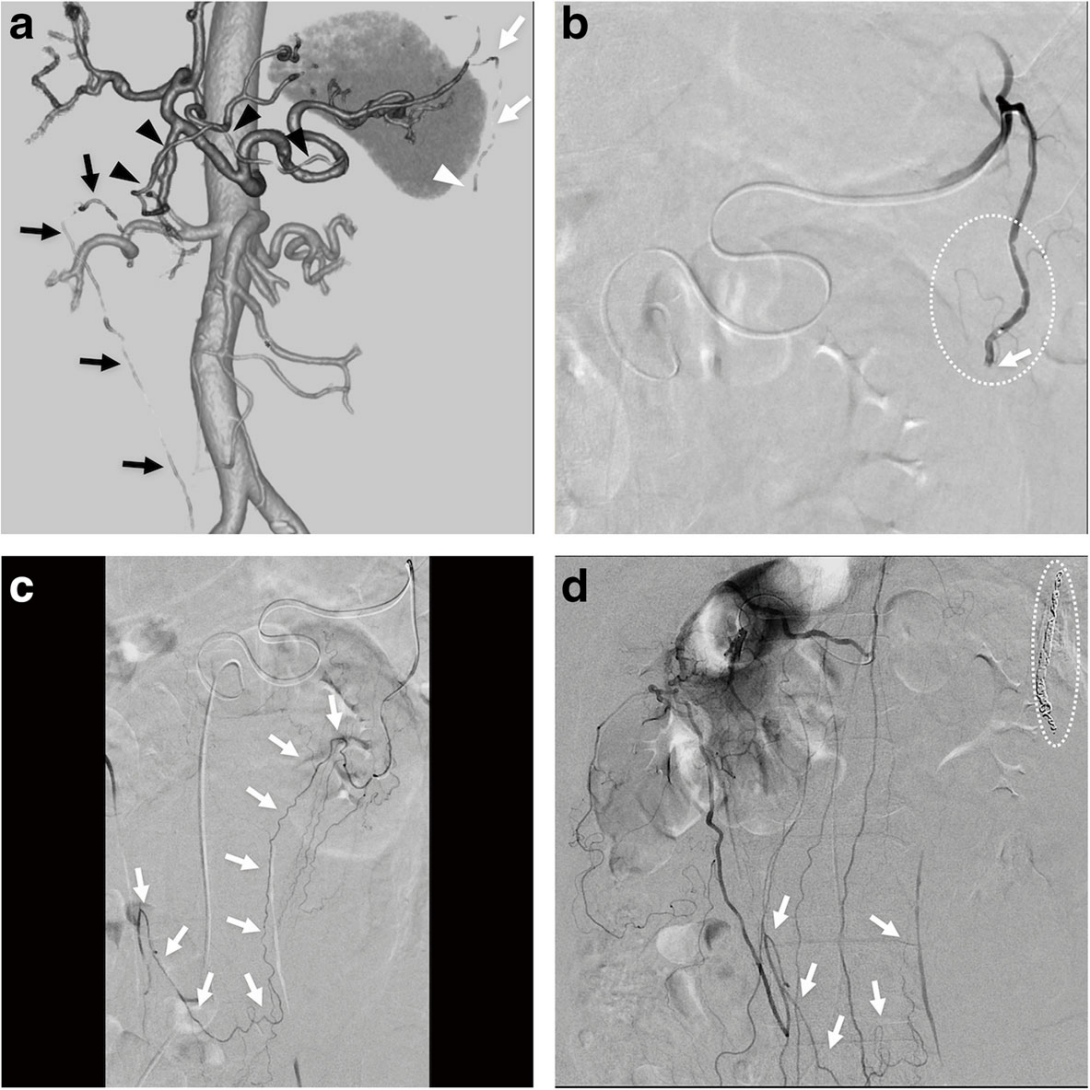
Images from a 60-year-old man with left OA bleeding associated with SAM. a Coronal volume-rendered computed tomography image in cranial and right-anterior projection reveals that a left OA (white arrows) arises acutely from a splenic artery branch and develops subtle dilation and stenotic change. The distal side of left OA is completely occluded (white arrowhead), presumably associated with SAM. Note that right gastroepiploic artery (black arrowheads) courses alongside the greater curvature of and one of the right omental branches off vertically (black arrows), which is found to be directly communicated with the to-be embolized left OA (white arrows). SAM is the most likely diagnosis, and transarterial embolization is planned. b DSA of left OA in right anterior oblique projection reveals that it acutely arises from a splenic artery and that it develops the dilation and stenotic change (dotted circle) and complete occlusion (arrow). Given that embolization of the proximal left OA at the arrow-indicating point could lead to incomplete hemostasis or rebleeding via arterial collateral network through another OA arising from right gastroepiploic artery, attempt was made to navigate the microcatheter into the distal side beyond the occluded point without complications. c DSA of the distal side of left OA in posteroanterior projection reveals that distal left OA does not develop findings suggestive of SAM. Note that the to-be-embolized left OA directly communicates with a right OA arising from right gastroepiploic artery (arrows). Embolization was successfully performed using isolation technique with coils. d DSA of gastroduodenal artery in posteroanterior projection reveals a right OA (arrows) directly communicating with the embolized left OA, which does not develop any further a potential bleeding point. Note that the SAM-associated left OA (dotted circle) is embolized with metallic coils. Complete hemostasis by isolation technique with coils is confirmed
